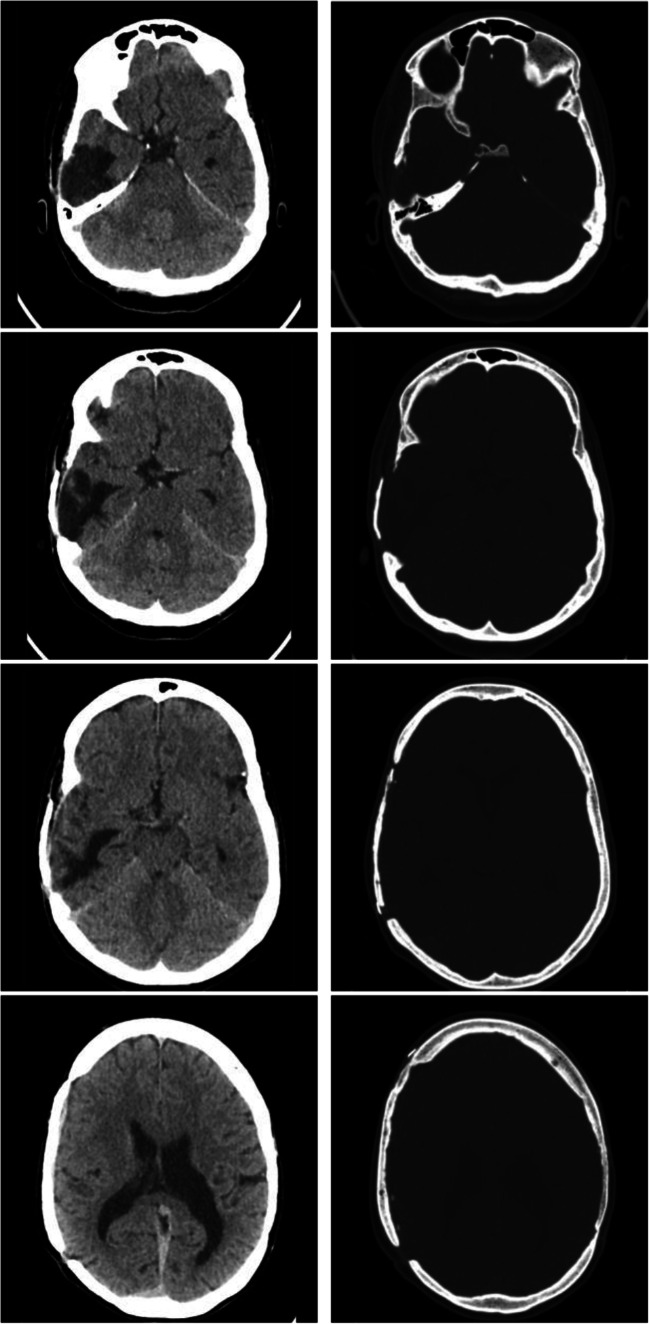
1-year radiological follow-up. The CT scan performed at 1 year from autologous bone flap replacement with different CT windows. On the left, the brain window shows the normal evolution of the right temporal intraparenchymal hemorrhage, with a better definition of a porencephalic area and resolution of any hyperdensity. On the right, the bone window highlights the autologous cranioplasty fixated with titanium plaques and screws to the calvaria
